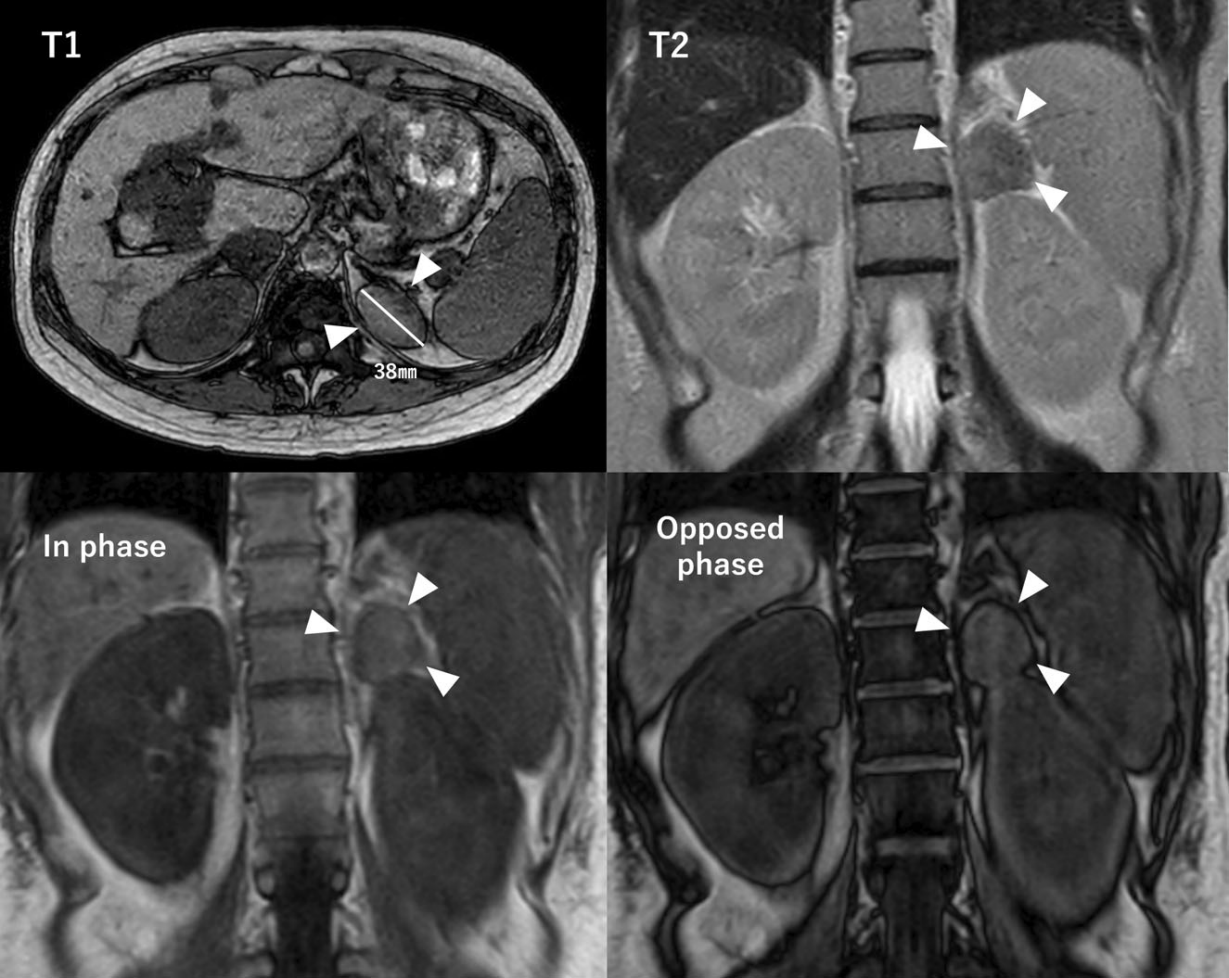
Magnetic resonance imaging on admission shows a left adrenal tumor with a long axis of 38 mm (arrowhead). Signal reduction was partially observed on opposed‐phase images, leading to diagnosis of cortical adenoma.
